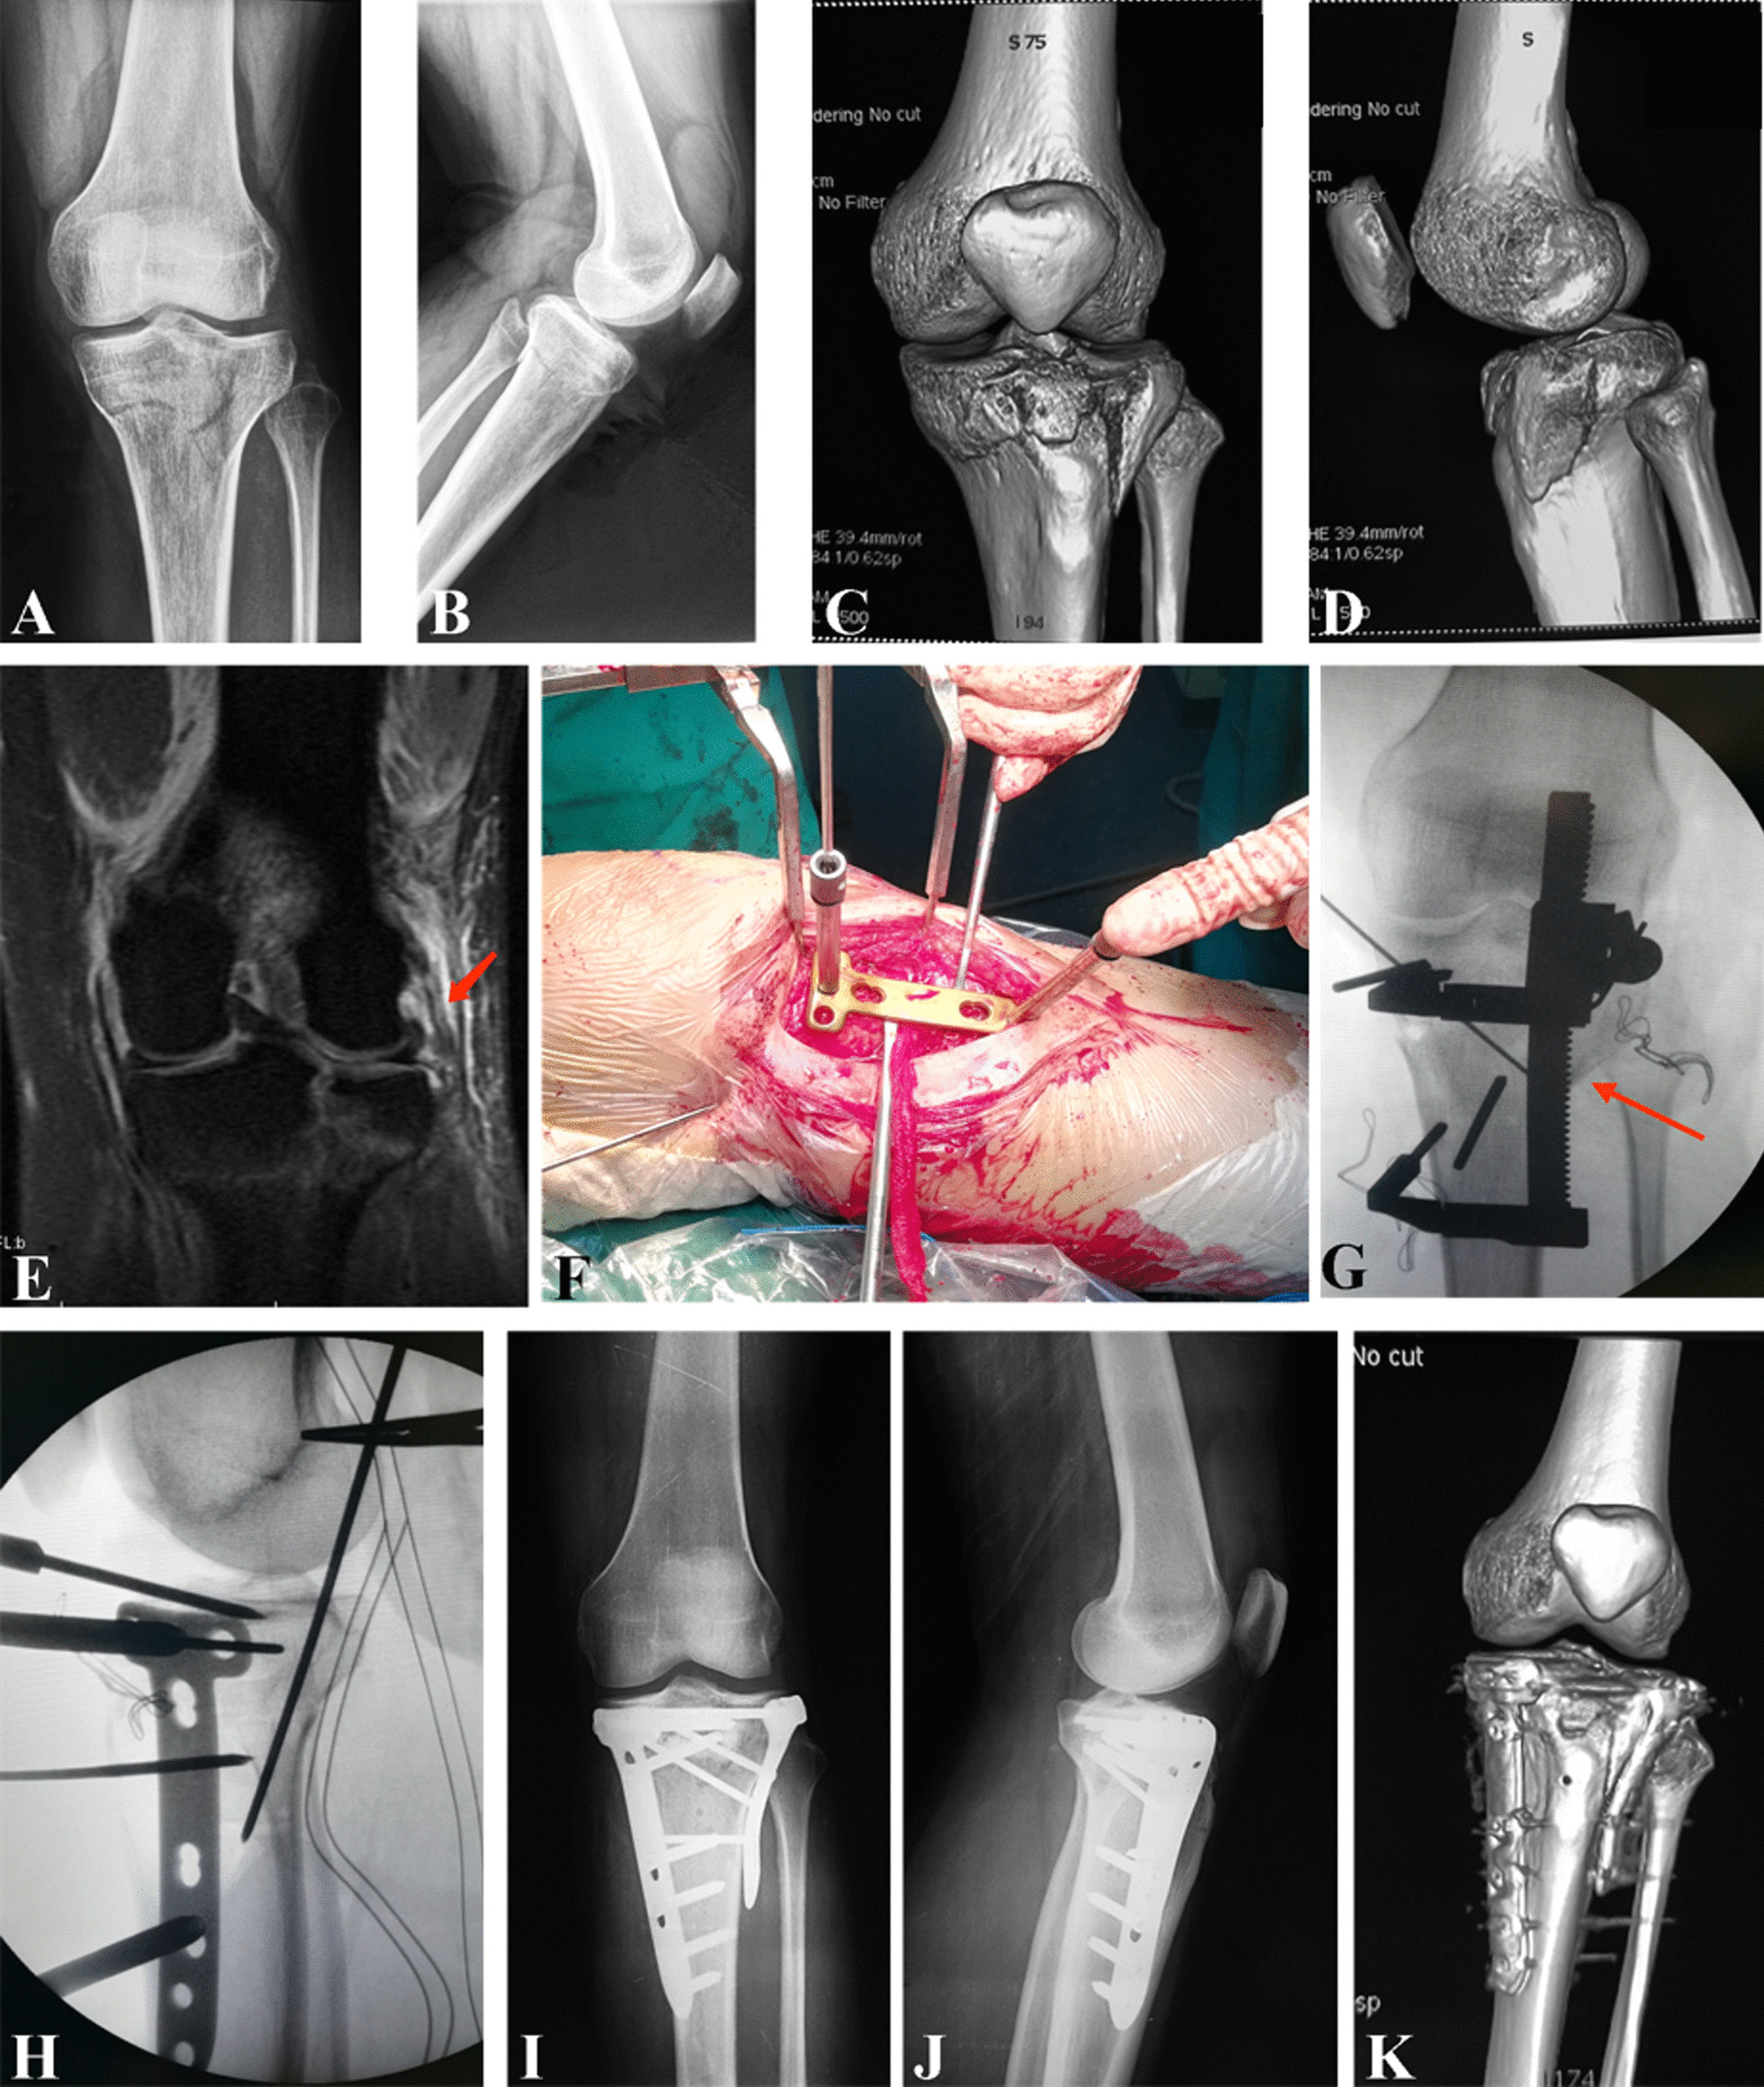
A 36-year old patient with hyperextension bicondylar fracture due to traffic accident. Radiological images of a 36-year-old Chinese female with HBTPF. a, b X-ray image of injured knee joint preoperatively. c, d 3D computed tomography reconstruction preoperatively. e intraoperative reduction and incisions. f, g fluoroscopy images of reduction on C-arm. Red arrow in f showed the retractor to reduce the compressed anterior plateau and maintain the reduction. h, i X-ray images within one week postoperatively. j, k 3D computed tomography reconstruction within 1 week postoperatively
